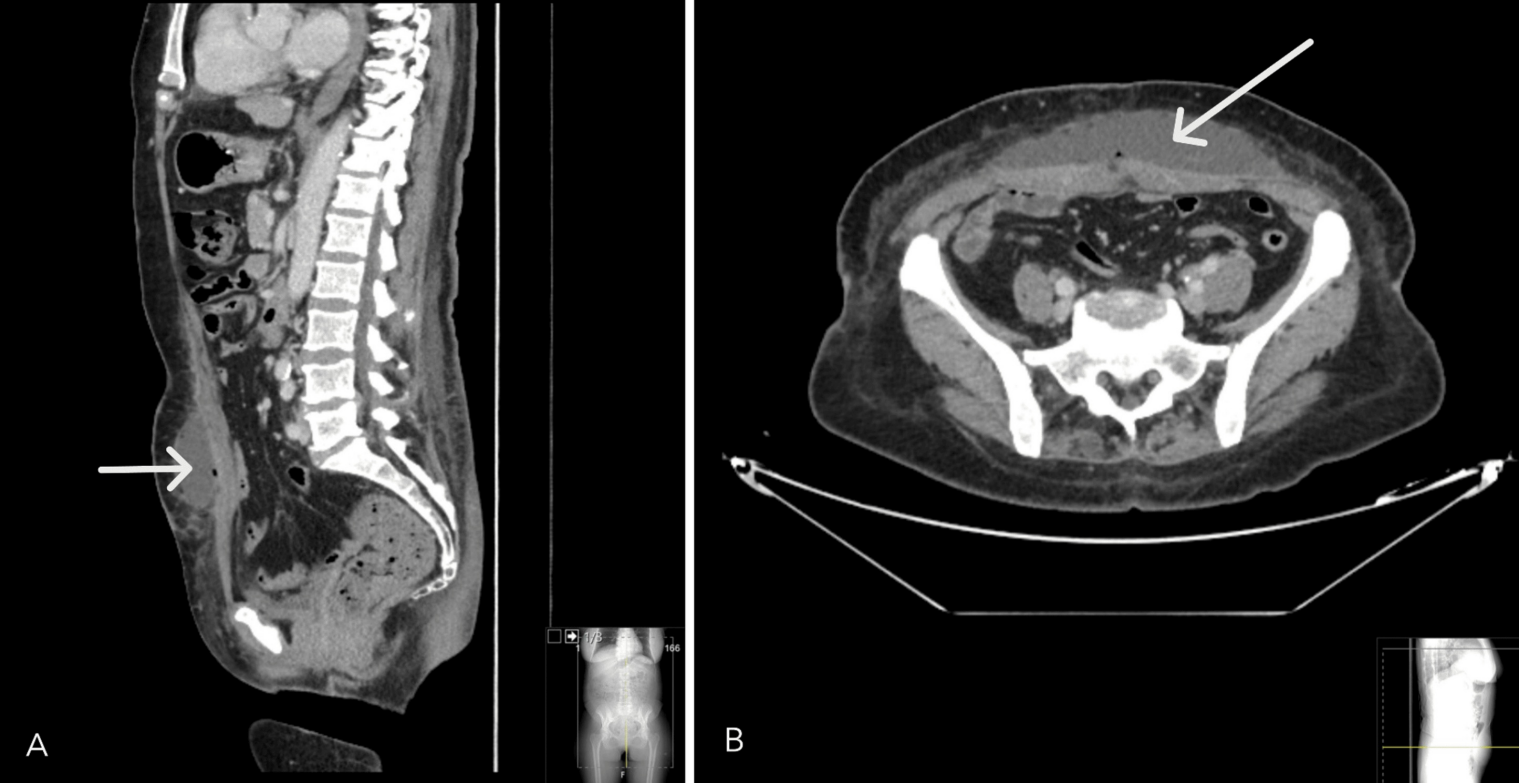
Abdominal CT scan: sagittal (A) and axial (B) views demonstrate a collection within the subcutaneous tissue of the anterior abdominal wall, containing fluid and gas, suggestive of an abscess (white arrows). CT - computed tomography
